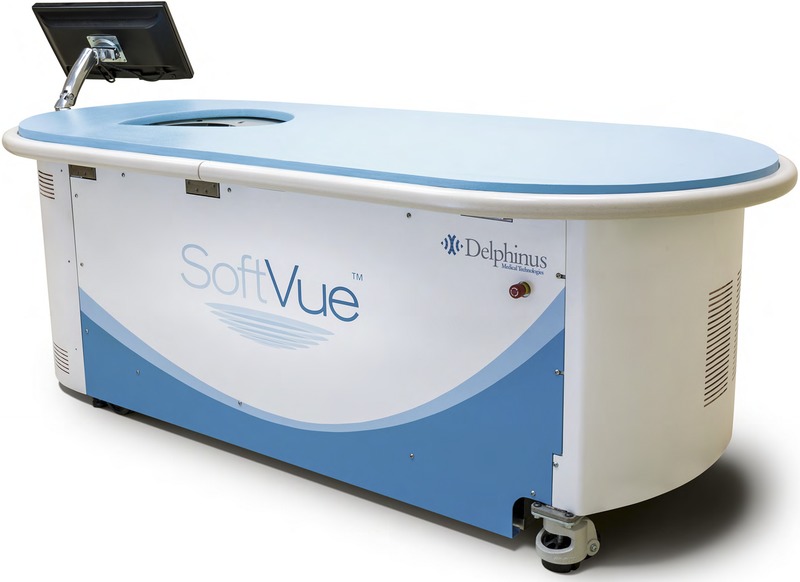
The SoftVue (Delphinus medical technologies) ultrasound tomography machine.
